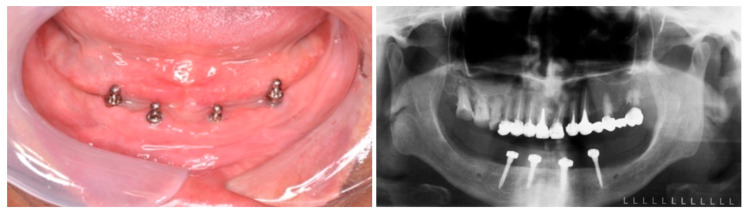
Clinical and radiographic positioning of mini dental implants.
